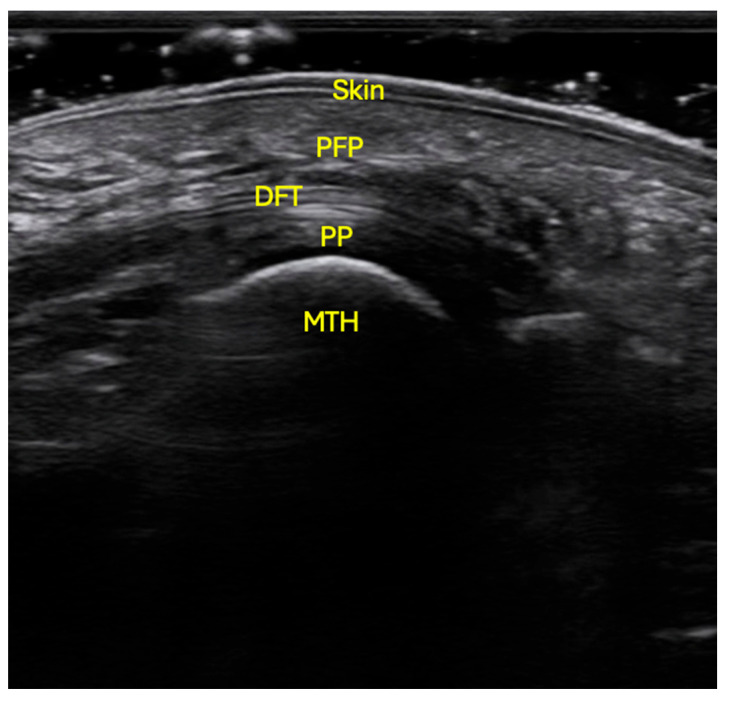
Ultrasonographic localization of the PFP at the level of the 2nd to 5th metatarsal heads. The probe was situated in longitudinal orientation, with no pressure, following the axis of the metatarsal, with the patient in prone position, flexed knee and neutral position of the ankle. Skin; PFP: plantar fat pad; DFT: digital flexor tendons; PP: plantar plate; MTH: metatarsal head.
